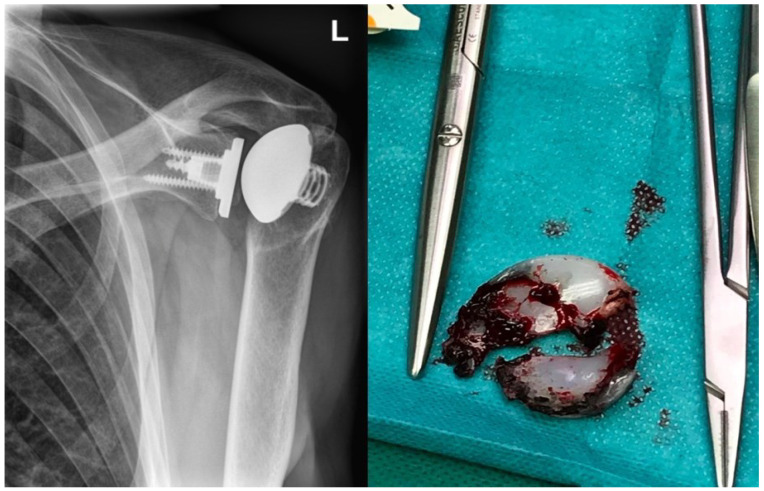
The image on the left side shows an X-ray taken shortly before a revision operation for conversion to RSA, demonstrating inlay abrasion with a tilt of the humeral head towards the metal-back as a direct sign of inlay abrasion and loosening of the humeral component with varus-tilt due to osteolysis and PE wear. The image on the right side presents an intraoperative situs of the inlay after removal.
